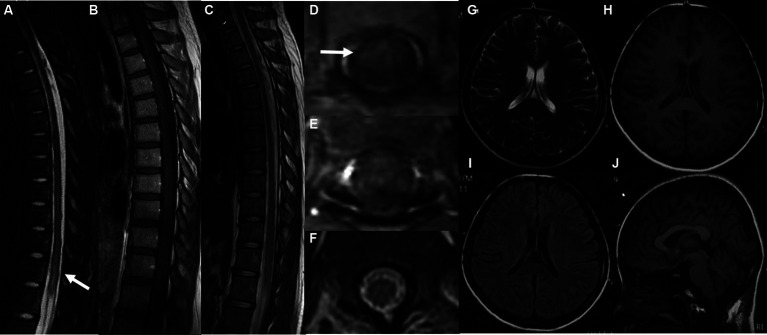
MRI images of the spinal cord both before and after therapy (A,C,D,F). Gadolinium-enhanced axial T1-weighted MRI of the spinal cord (B,E). MRI of the brain (G–J) revealed the absence of brain lesions. The sagittal T1-weighted image (A) and axial T1-weighted image (D) of the thoracic spinal cord before therapy revealed an abnormal signal in the thoracic and lumbar spinal cord (T11-L1). We did not observe obvious enhancement of spinal lesion in enhanced T1-weighted MRI (B,E). After therapy, the sagittal T1-weighted image (C) and axial T1-weighted image (F) demonstrated a significant reduction in the size of the previously observed lesions.
